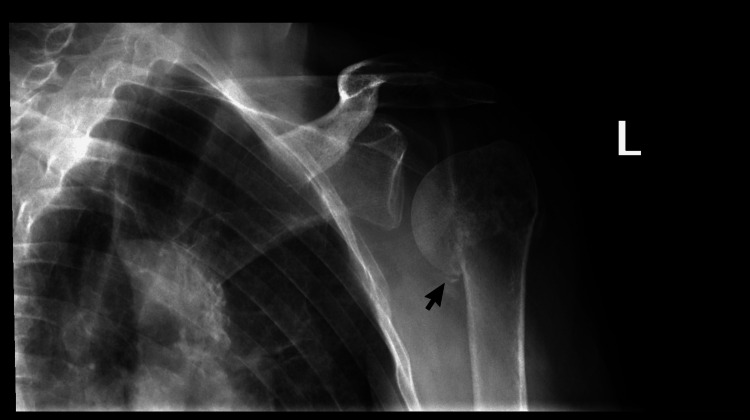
X-ray imaging showcasing fracture of the surgical neck of the left humerus
